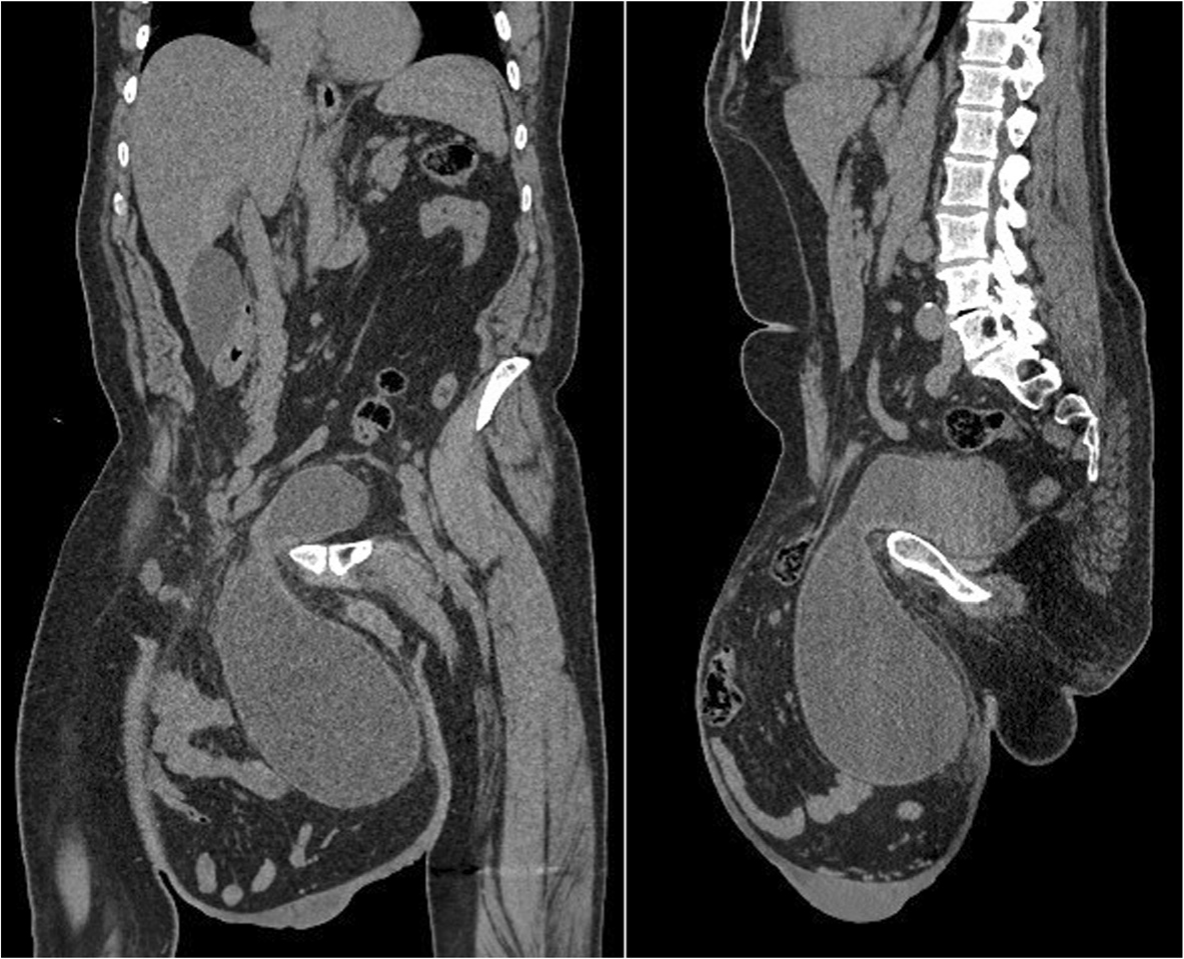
Computed tomography findings. Coronal and sagittal sections show urinary bladder and intestinal segments incarcerated in the large inguinoscrotal hernia sac
